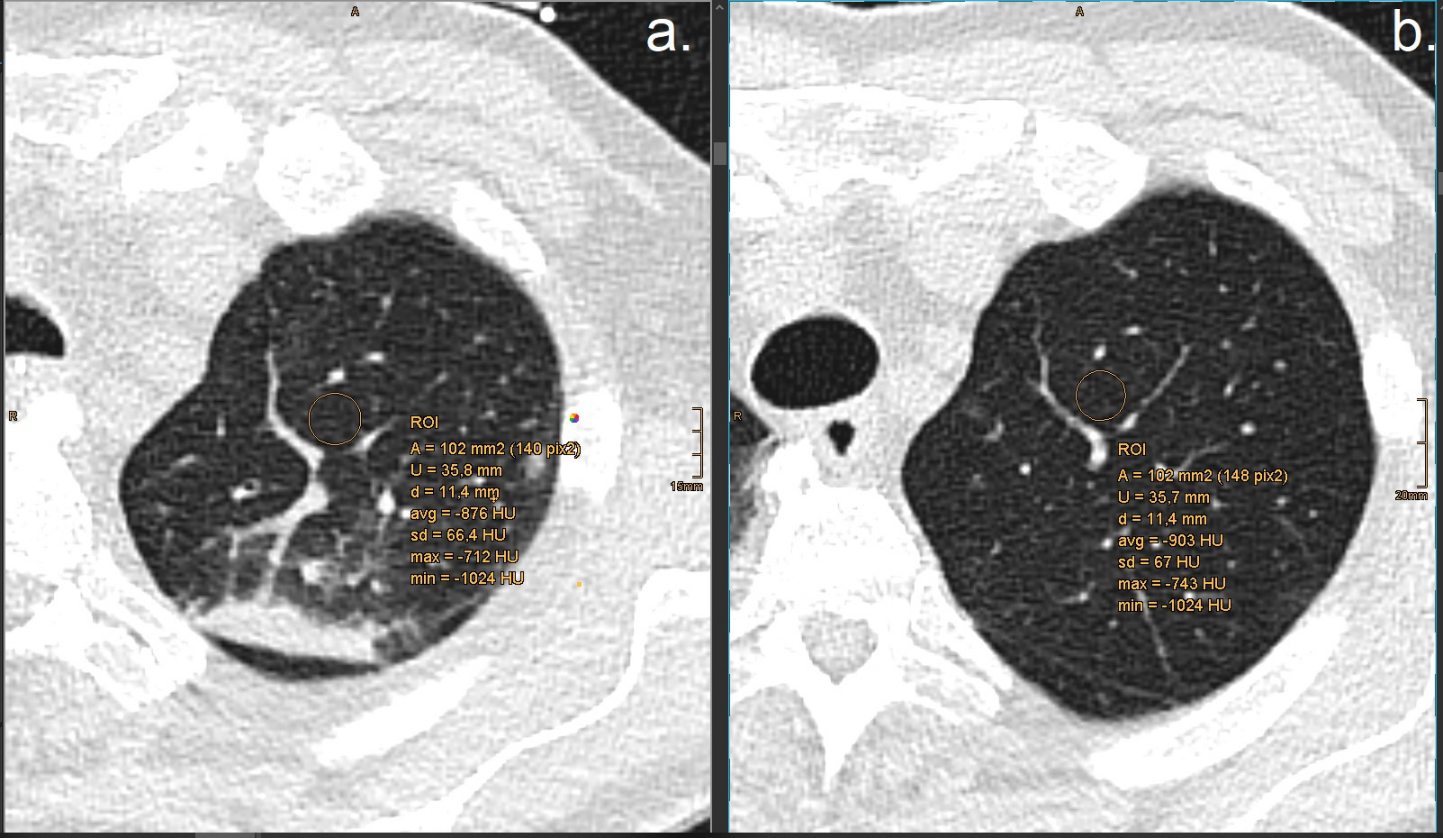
ROI measurements. Manual ROI measurements in the left upper lobe of a 45-year-old woman with COVID 19 pneumonia at initial CT scan (a.) and at follow-up CT scan after 45 days (b.), a decreasing in attenuation values by -27 HU can be seen between initial scan and follow-up.
